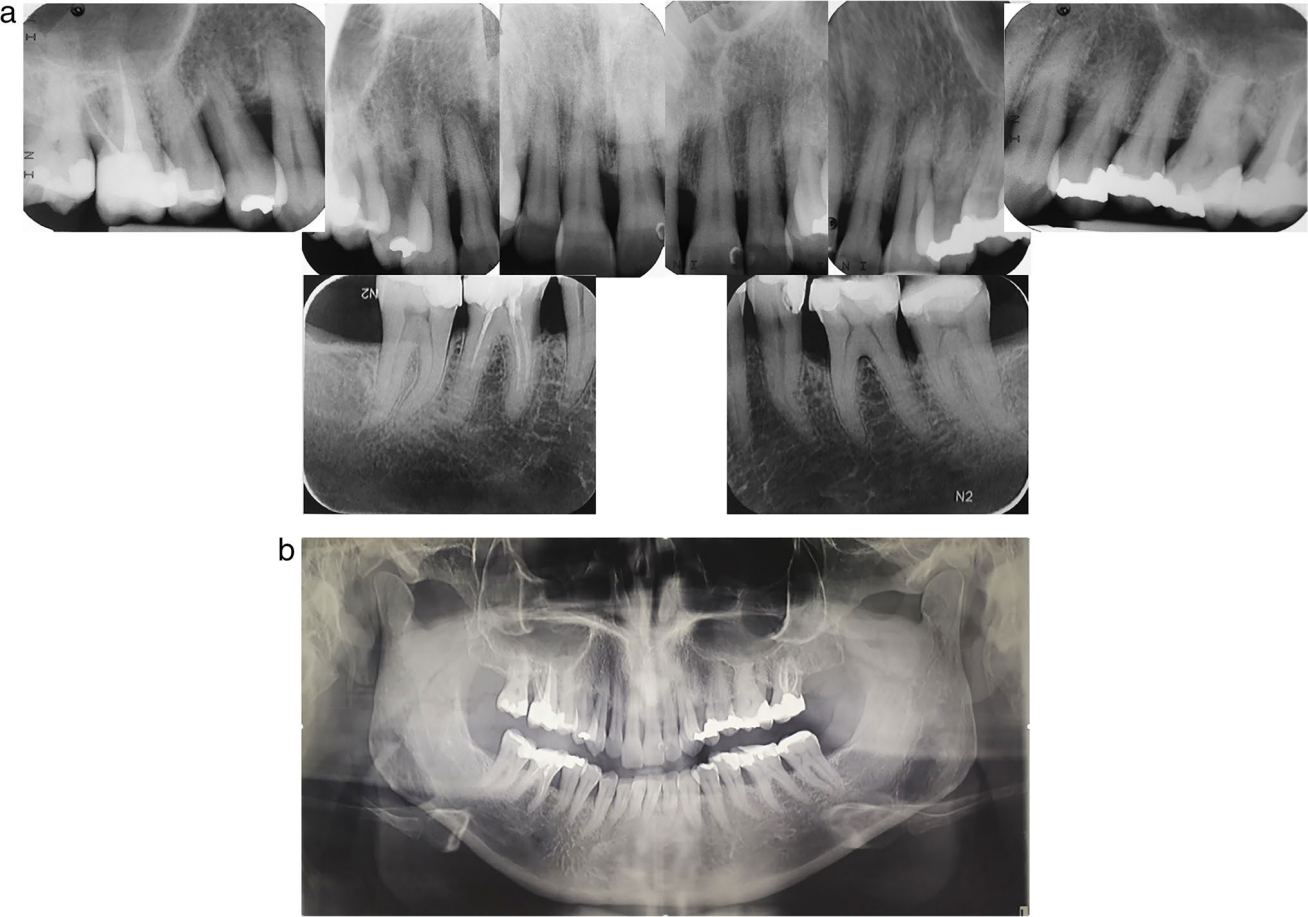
a Local radiographs of a representative patient. b Orthopantomogram of a representative individual
